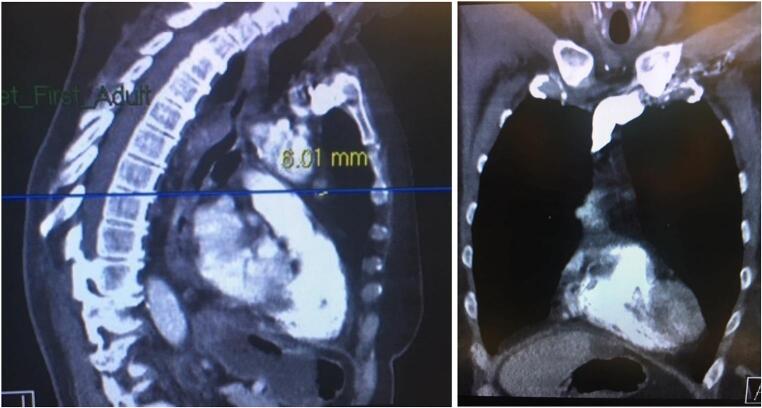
CT scan showing mass in the anterior mediastinum, below the angle of Louis.
